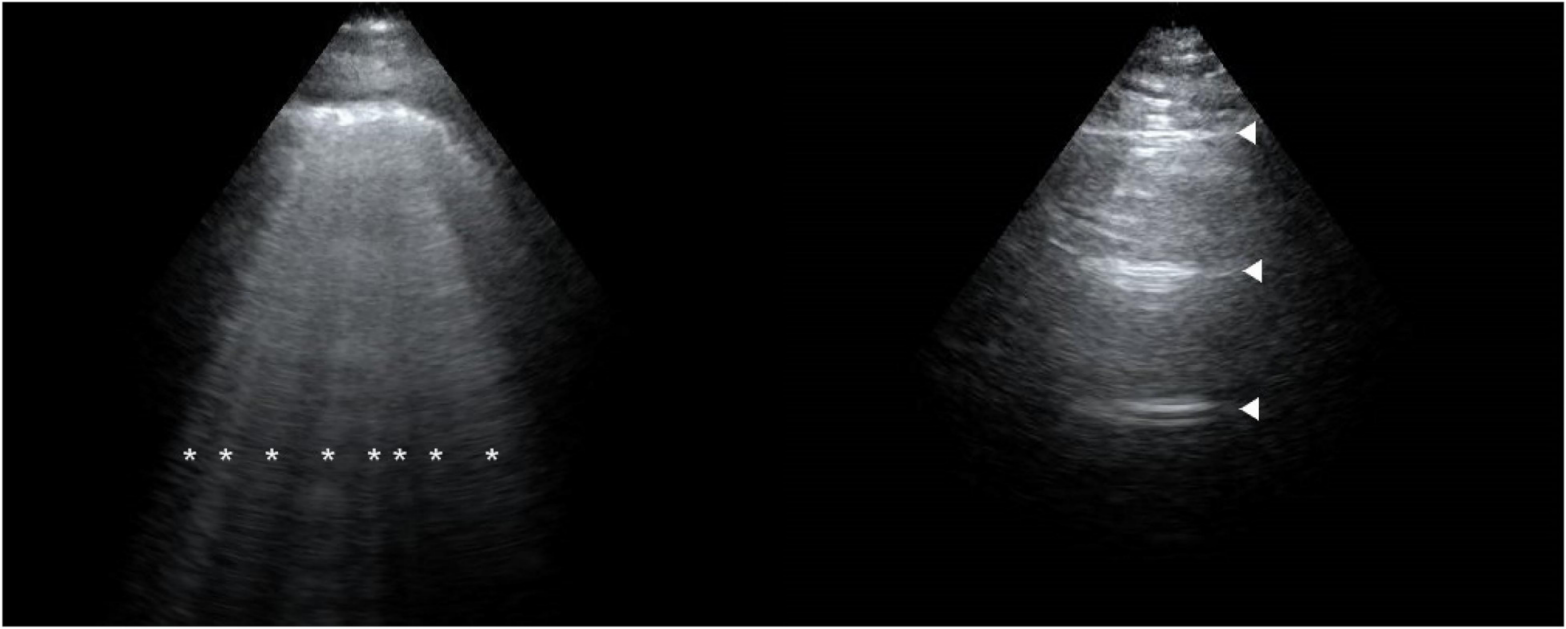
Lung ultrasound performance of a preeclampsia patient (left) and a healthy patient (right) at 2 h postpartum. The image of a preeclampsia patient is filled with B-lines (*). The image of a healthy patient is shown as A-lines (▴).
